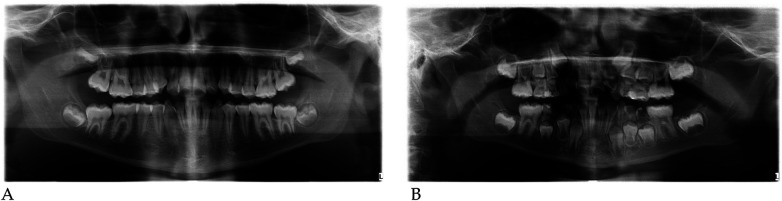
Pre- (A) and post-treatment (B) panoramic radiographs of case 12.
